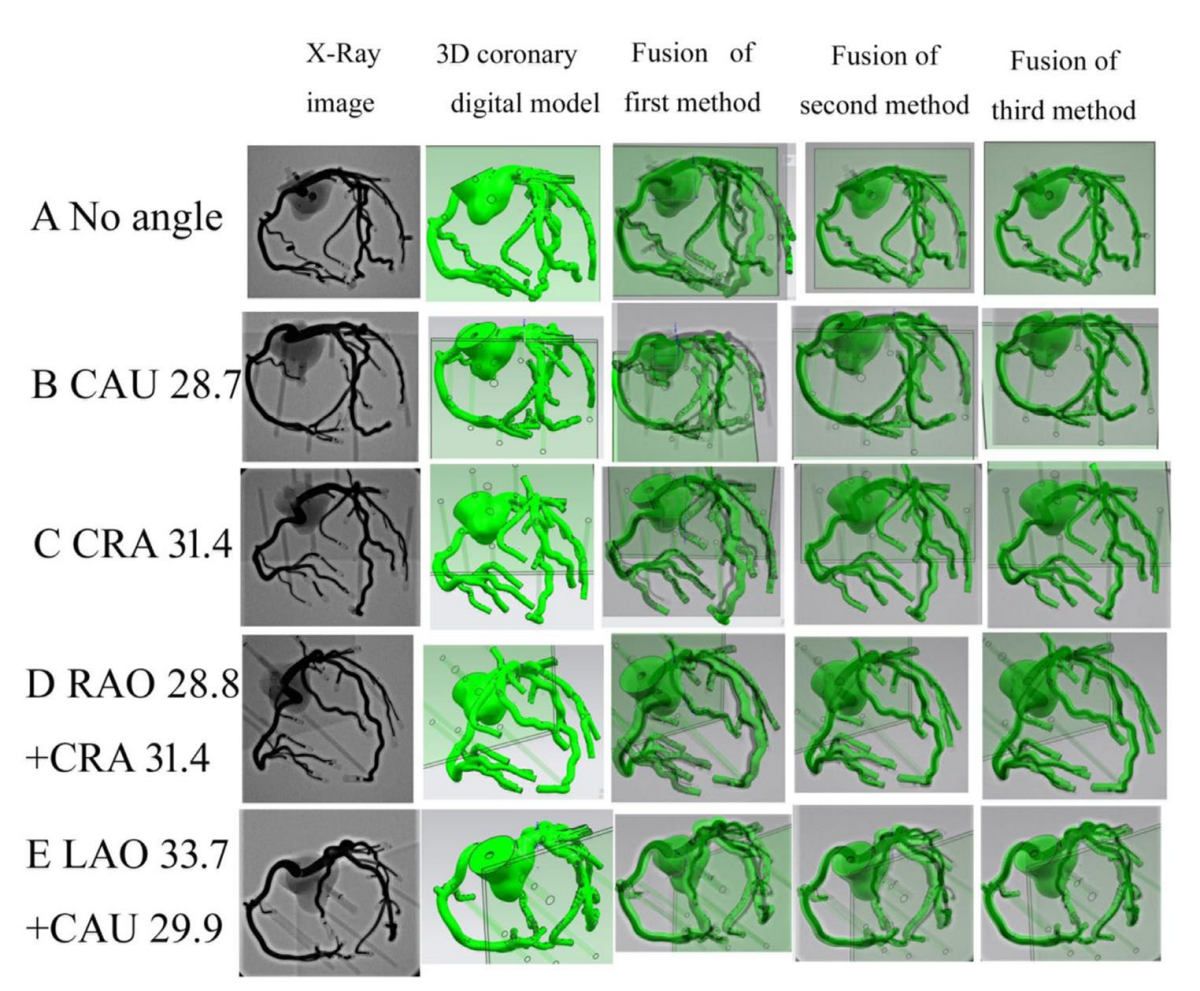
Effects of using three different methods to process the coronary artery digital model projection for image fusion. First method: perspective projection with the detector as viewpoint. Second method: parallel projection. Third method: mirror image of perspective projection with the X-ray tube as the viewpoint. CAU: caudal; CRA: cranial; RAO: right anterior oblique; LAO: left anterior oblique
